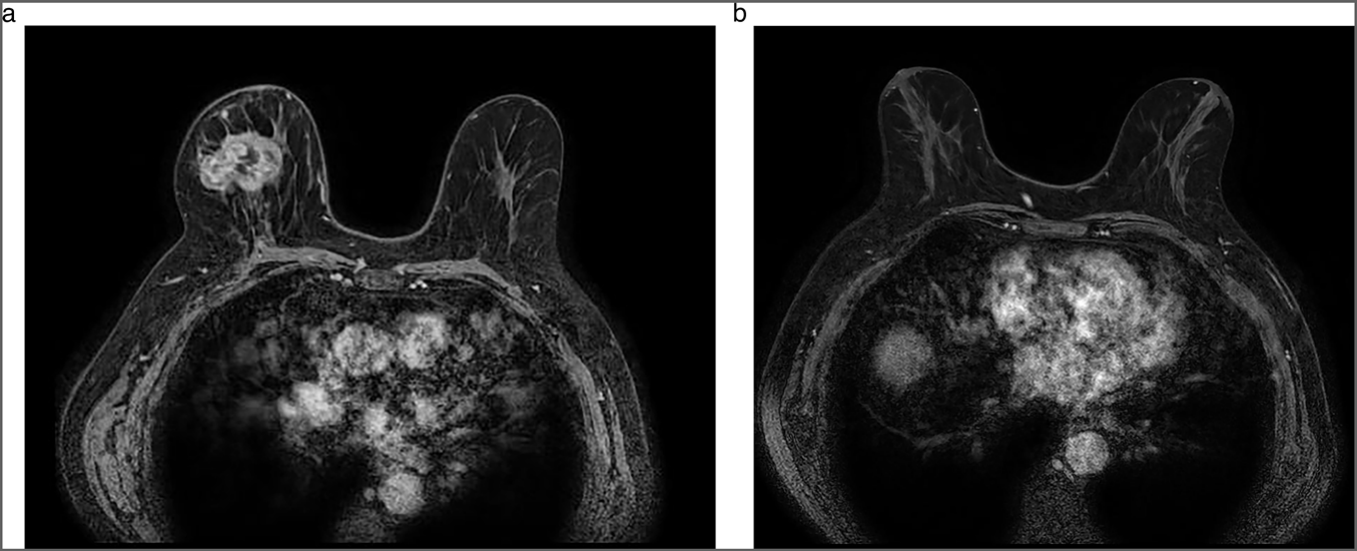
Example of a 77-year-old woman diagnosed with a right unifocal invasive breast carcinoma grade 3 with a triple-negative subtype and biopsy-proven axillary lymph node metastases (cN1). Baseline breast MRI prior to NACT demonstrated a 47 mm large mass (a). Post-NACT breast MRI demonstrated the achievement of a breast radiologic complete response (b). Following NACT and surgery, the patient achieved ypT0N0. NACT, neoadjuvant chemotherapy
